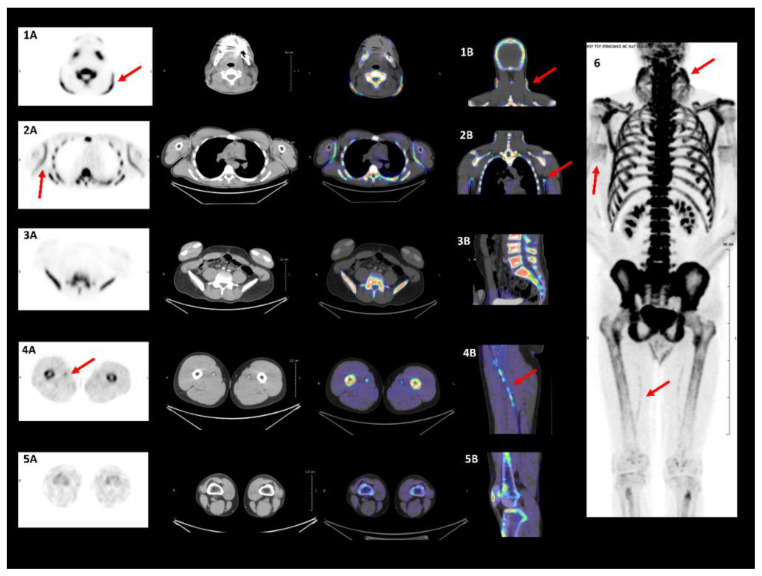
18F-NaF uptake in specific damaged skin/arteries in PXE. Damaged skin in neck (arrows (1A,1B)) and axillary folds (arrows (2A,2B)) vs healthy skin in lumbar region (3A,3B) on PET/CT imaging. Molecular calcification targeted with 18F-NaF. Damaged femoral arteries on PET/CT imaging. Molecular calcification targeted via PET/CT demonstrating 18F-NaF femoral arteries wall uptake (arrows (4A,4B)). 18F-NaF uptake absent in popliteal arteries walls (5A,5B). Maximum intensity projection indicates damaged vascular and skin sites (arrow 6).
